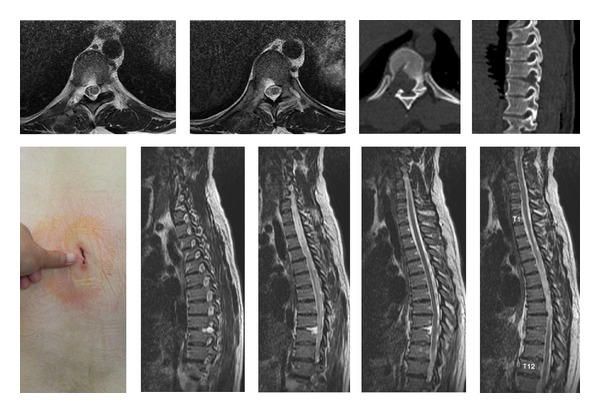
Postoperative CT, MRI images of Case 5. View of the incision.
